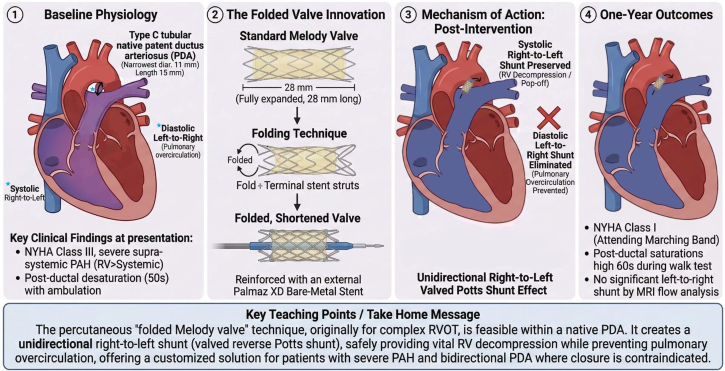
Infographic Detailing the Percutaneous Creation of a Valved Reverse Potts Shunt Using a Folded Melody Valve Within a Native Patent Ductus Arteriosus The summary outlines the patient's baseline physiology, the valve modification technique, the unidirectional hemodynamic mechanism of action, and successful 1-year clinical outcomes. MRI = magnetic resonance imaging; PAH = pulmonary arterial hypertension; PDA = patent ductus arteriosus; RV = right ventricle; RVOT = right ventricular outflow tract.
